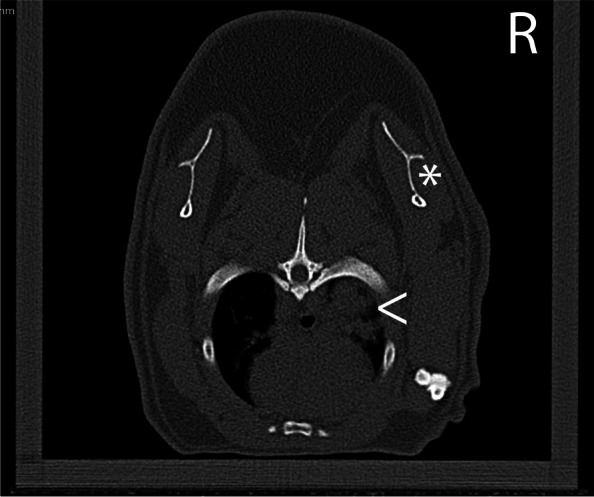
Computed tomography image from case #2. Transverse image at the level of the mid-scapula (*). Within the dorsal aspect of the right cranial lung lobe there are areas of pulmonary consolidation with relatively well-defined margins. ( <) The patient is contained in a fitted box. Image displayed C:320 W2800. Slice thickness 0.69 mm, 120 kV, 139 mA. The pulmonary changes could represent mycobacteriosis as the pulmonary parenchyma was positive on PCR. The distribution of the consolidation, the timeframe of event, and the evolution of the consolidation on the repeat CT would not be considered usual for either aspiration pneumonia nor pulmonary atelectasis
